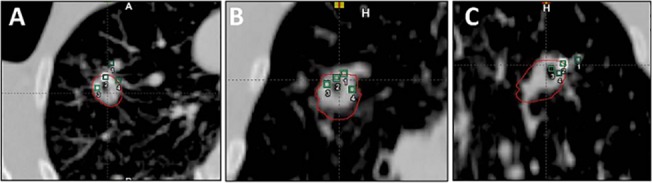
CBCT images of 4 fiducials placed in or near a tumor in the right upper lobe treated with SBRT in (A) axial, (B) frontal, and (C) sagittal views. The four green squares indicated the four fiducials identified on the planning CT.
